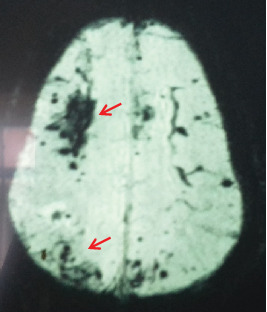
MRI/SWI image of the brain. There is a significant blooming with multiple peripheral and central bleeding foci in the cerebral hemispheres (arrow).
